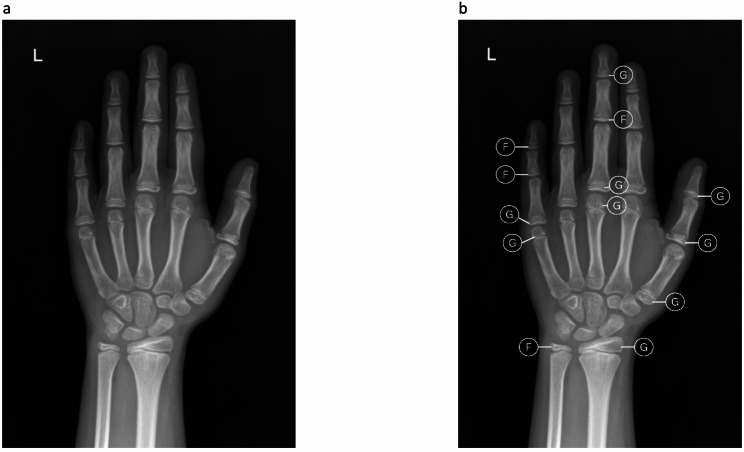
Hand radiograph of a female child. (a) The GP method matched the radiograph to the 11-year atlas standard. (b) TW3-RUS yielded a bone age of 10 years 9 months based on individual bone staging and cumulative scoring
